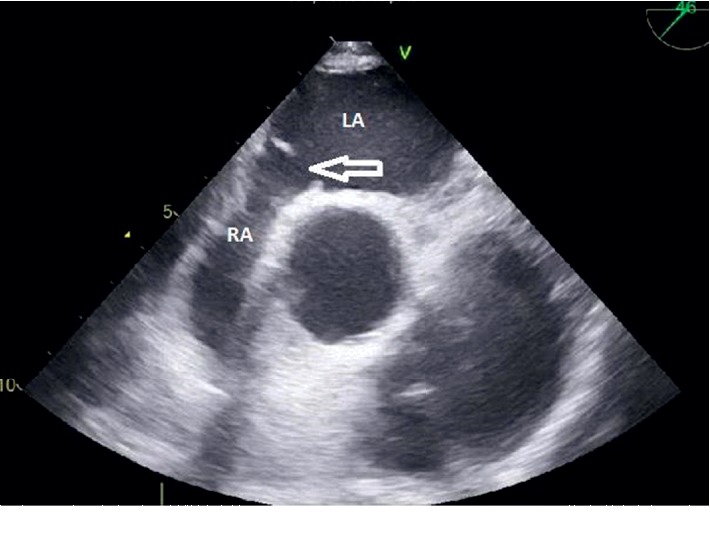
Transesophageal echocardiogram: midesophageal aortic valve short axis view of atrial septal defect. RA: right atrium, LA: left atrium.
